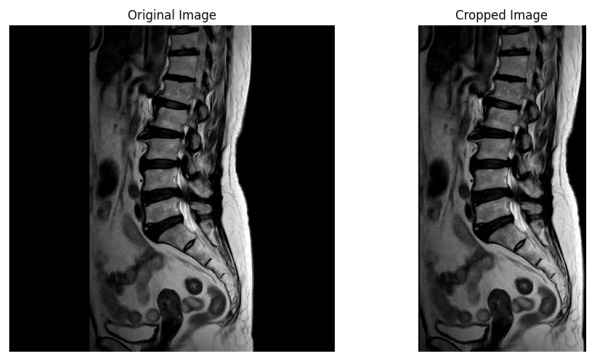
Preprocessing step showing the original MRI image and the automatically cropped image used for subsequent analysis.
